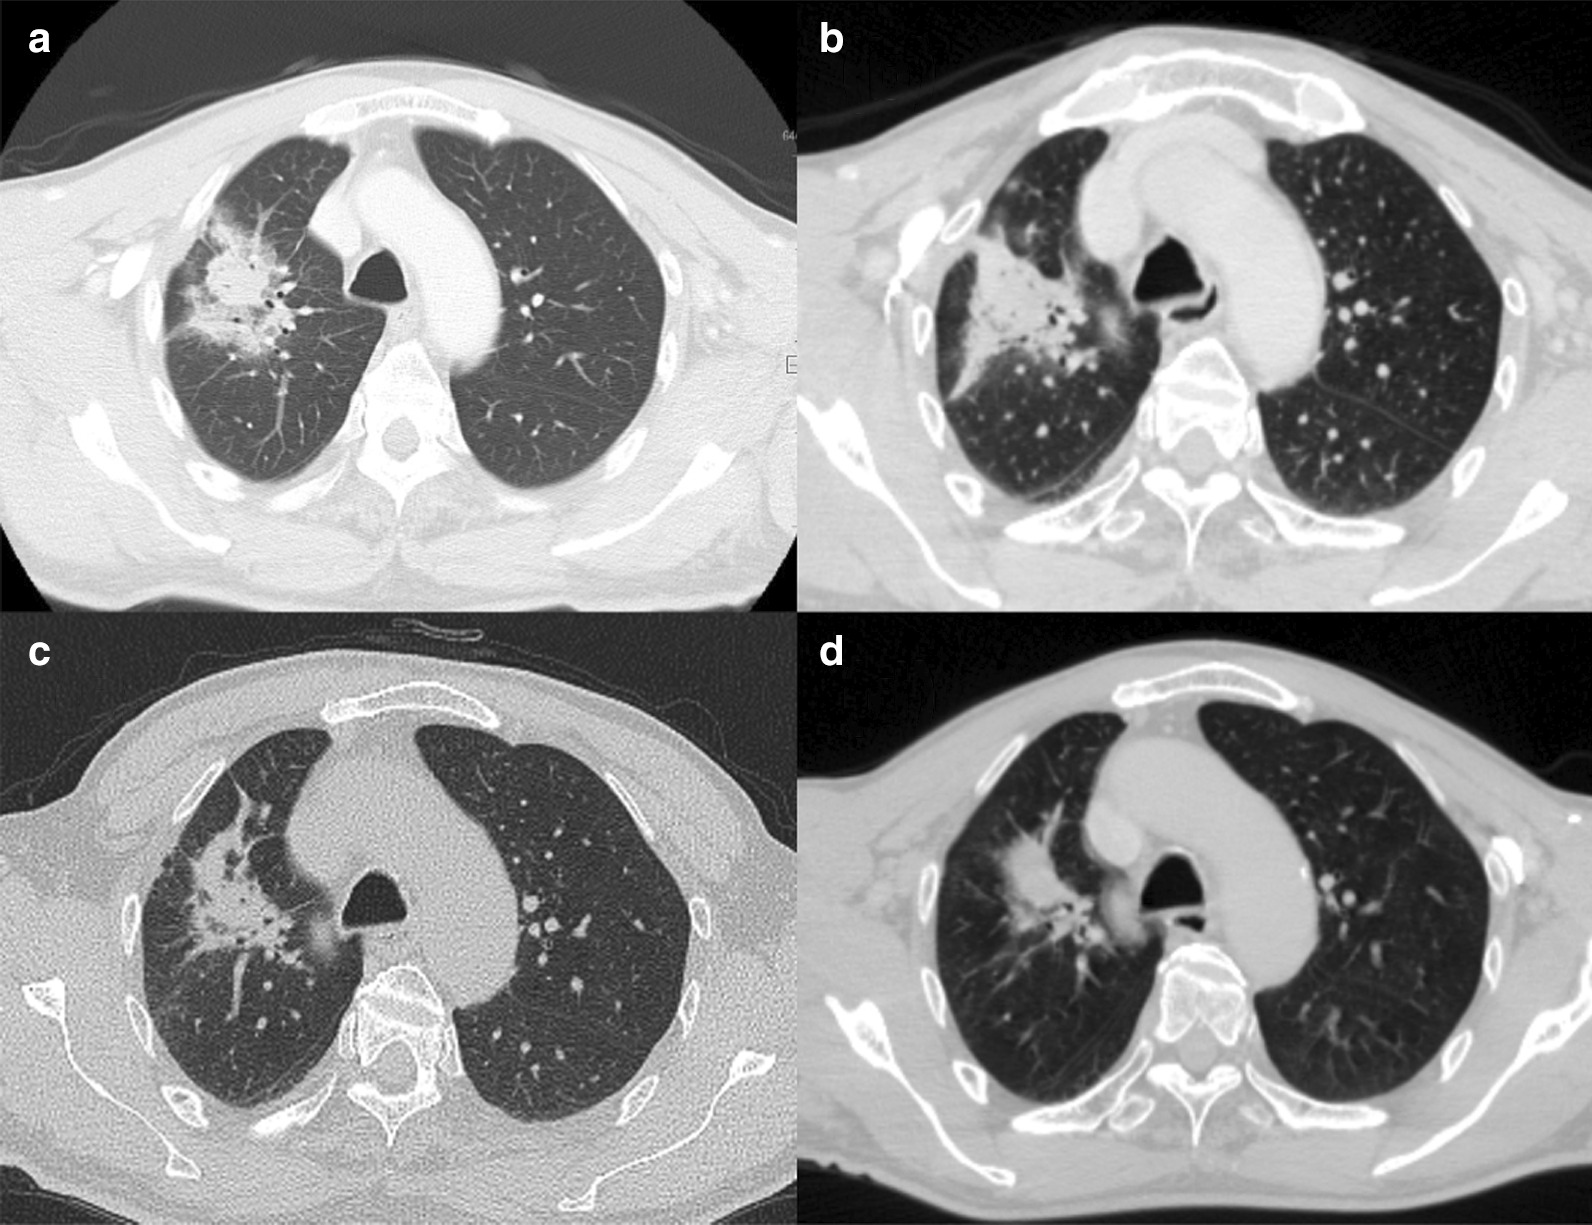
Radiographical course of radiation pneumonitis developed after CyberKnife treatment of the right upper lung tumor. The extent of radiation pneumonitis was limited and it has remained stable for 21 months. a 3 months, b 6 months, c 15 months, and d 21 months after CyberKnife
